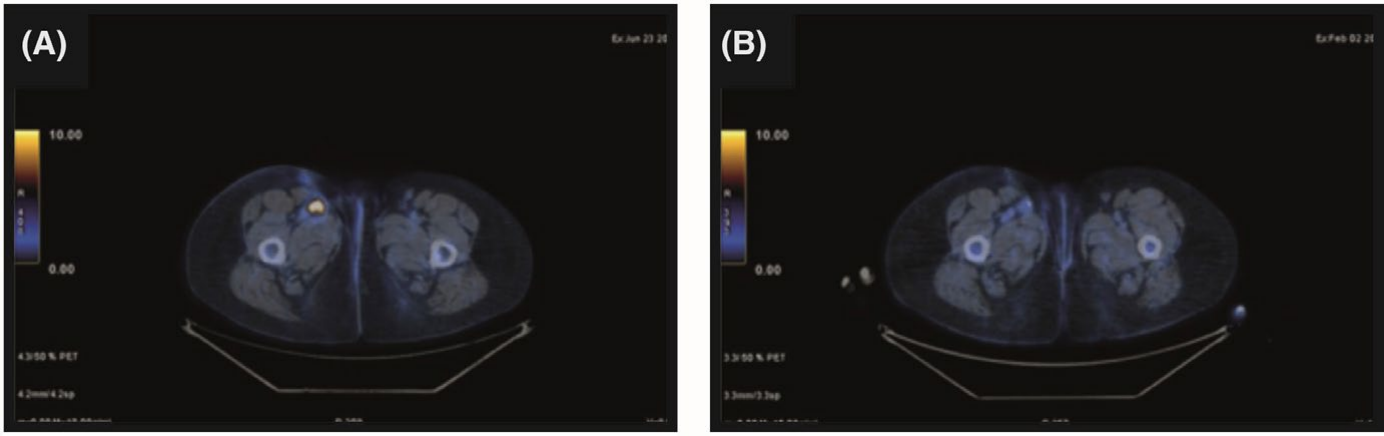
18F‐deoxyglucose positron emission tomography (FDG‐PET) conducted pretherapy demonstrating uptake in the right inguinal nodes (A) and after 7 mo of therapy, demonstrating substantial metabolic normalization in the inguinal nodes (B)
